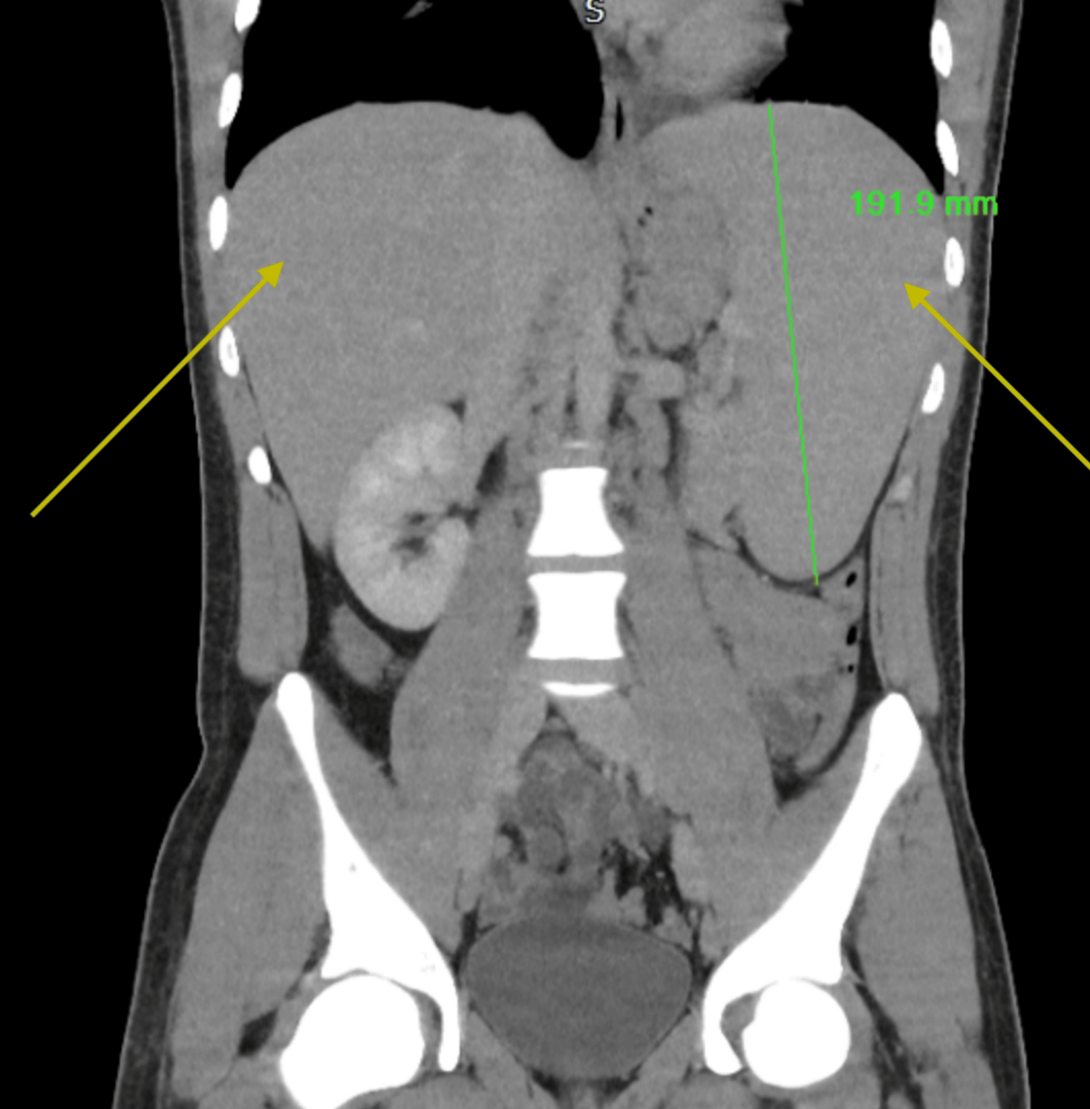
CT of the abdomen demonstrating hepatosplenomegaly CT: computed tomography
